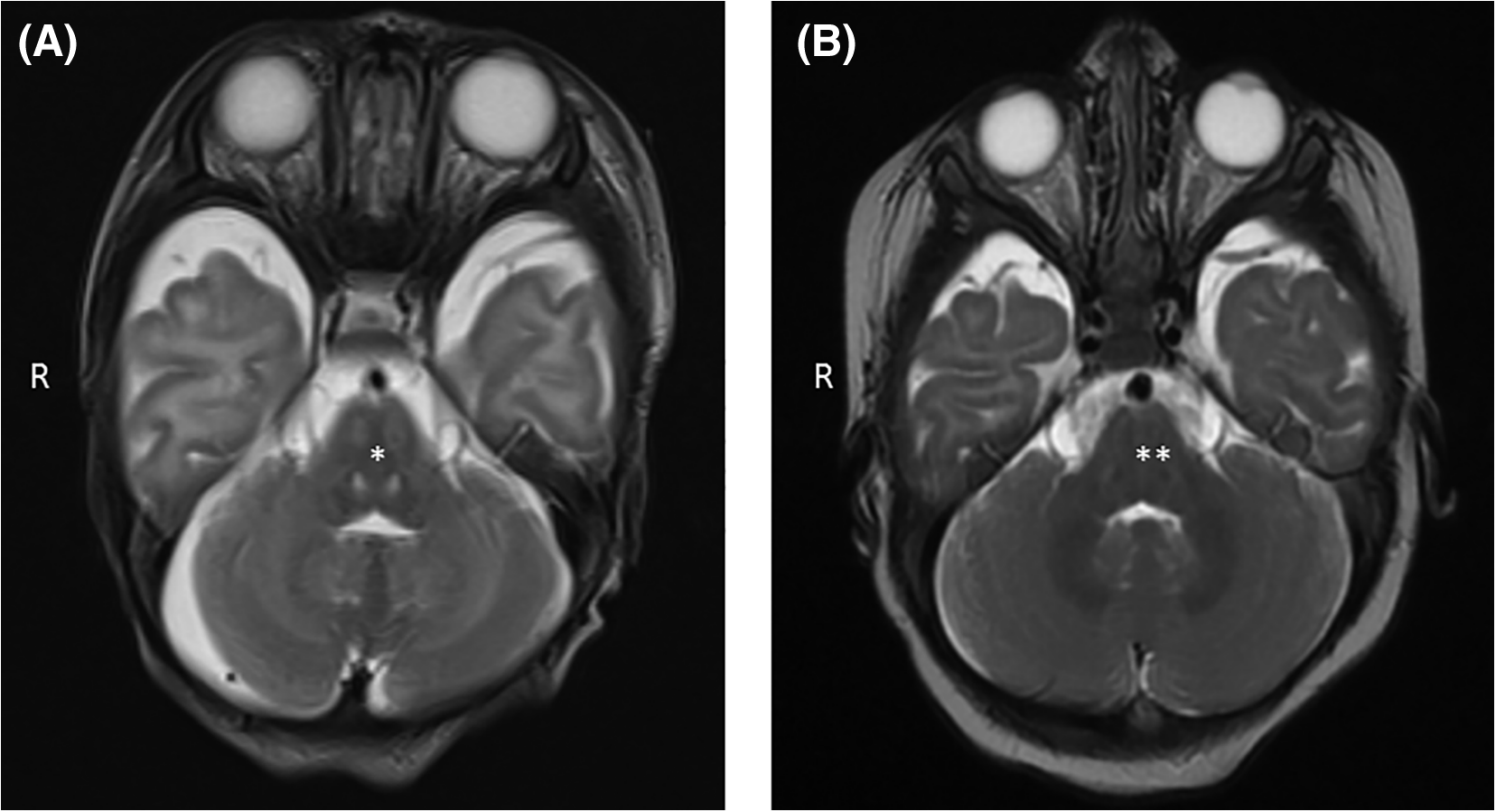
Magnetic resonance imaging brain in a 6‐week‐old baby with hypermethioninemic encephalopathy showing increased T2 signal intensity in the pons (A, *) and normalization 4 months later (B, )
